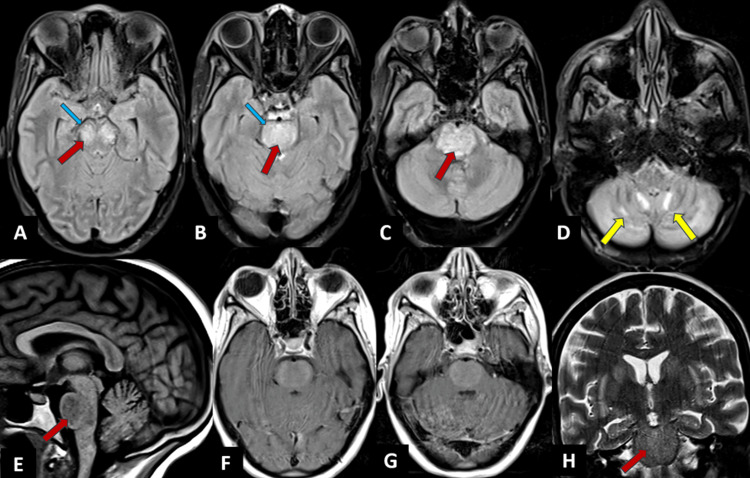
Pontine and extrapontine involvement in ODS Axial FLAIR images (A-C), sagittal T1-weighted image (E), and coronal T2-weighted image (H) demonstrate a symmetric central pontine lesion, appearing hyperintense on T2-weighted and FLAIR images and hypointense on T1-weighted imaging (red arrows), with relative sparing of the peripheral fibers (blue arrows). Post-contrast images (F, G) demonstrate faint patchy enhancement within the lesion. Axial FLAIR image (D) demonstrates bilateral extrapontine cerebellar lesions (yellow arrows). FLAIR: fluid-attenuated inversion recovery; ODS: osmotic demyelination syndrome
